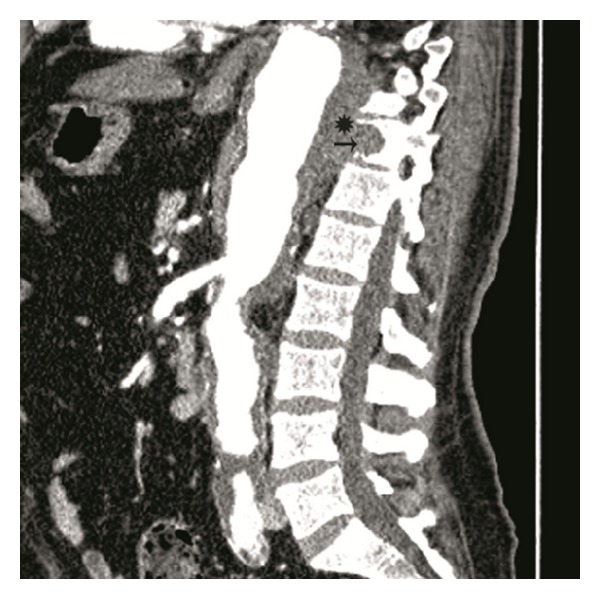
Contrast-enhanced multidetector CT angiography lateral MPR image shows chronic-contained aneurysm rupture (*) of the aorta with vertebral erosion and lysis (arrow).
